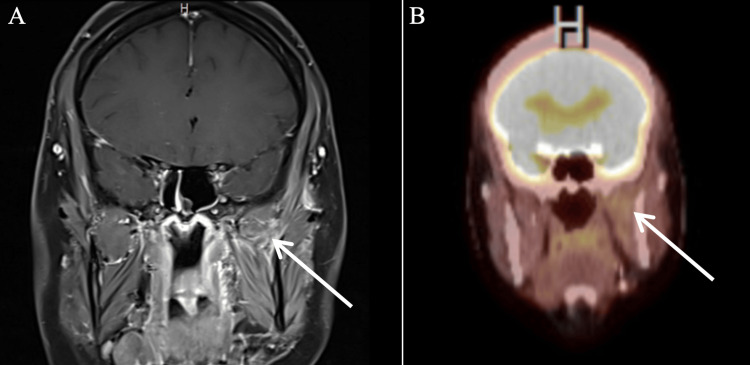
Three-month post-chemoradiation imaging demonstrating a persistent soft tissue density within the left masticator space on T1 coronal MRI of the face/neck/orbit (A). This area did not have FDG avidity upon concurrent PET/CT (B) FDG: fluorodeoxyglucose
